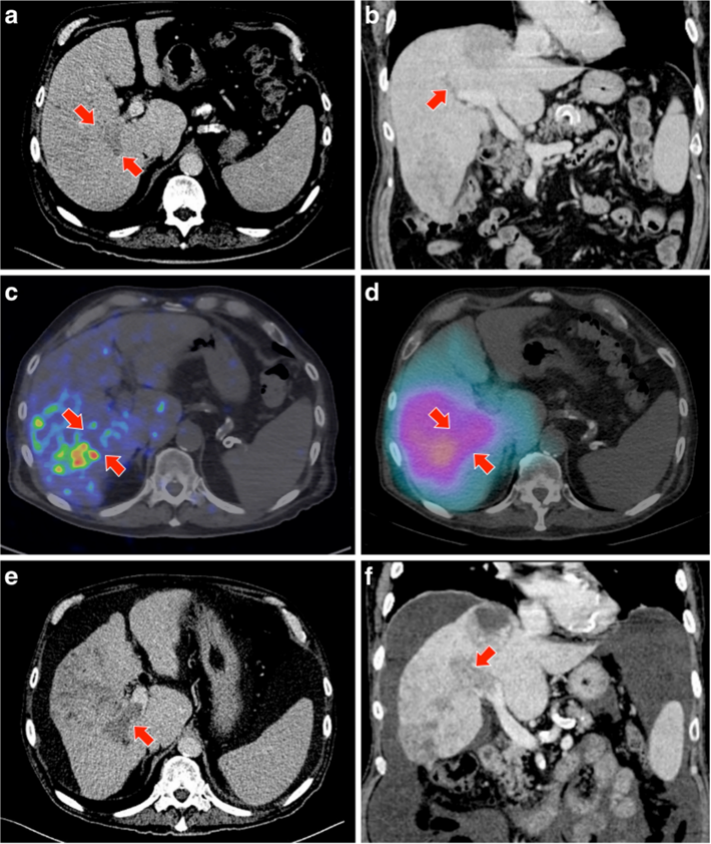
Patient 12. (a, b) Portal vein tumor thrombosis (arrow) in a multifocal HCC seen on portal venous phase of a triphasic CT liver. (c)^90^Y PET/CT demonstrates absent activity within the portal vein tumor thrombus. (d) The diffuse nature of ^90^Y bremsstrahlung SPECT/CT is unable to resolve the absence of activity within the portal vein tumor thrombus. (e, f) Four months post-radioembolization, a follow-up triphasic CT liver in the portal venous phase shows significant progression of portal vein tumor thrombosis, validating the ^90^Y PET/CT findings.
